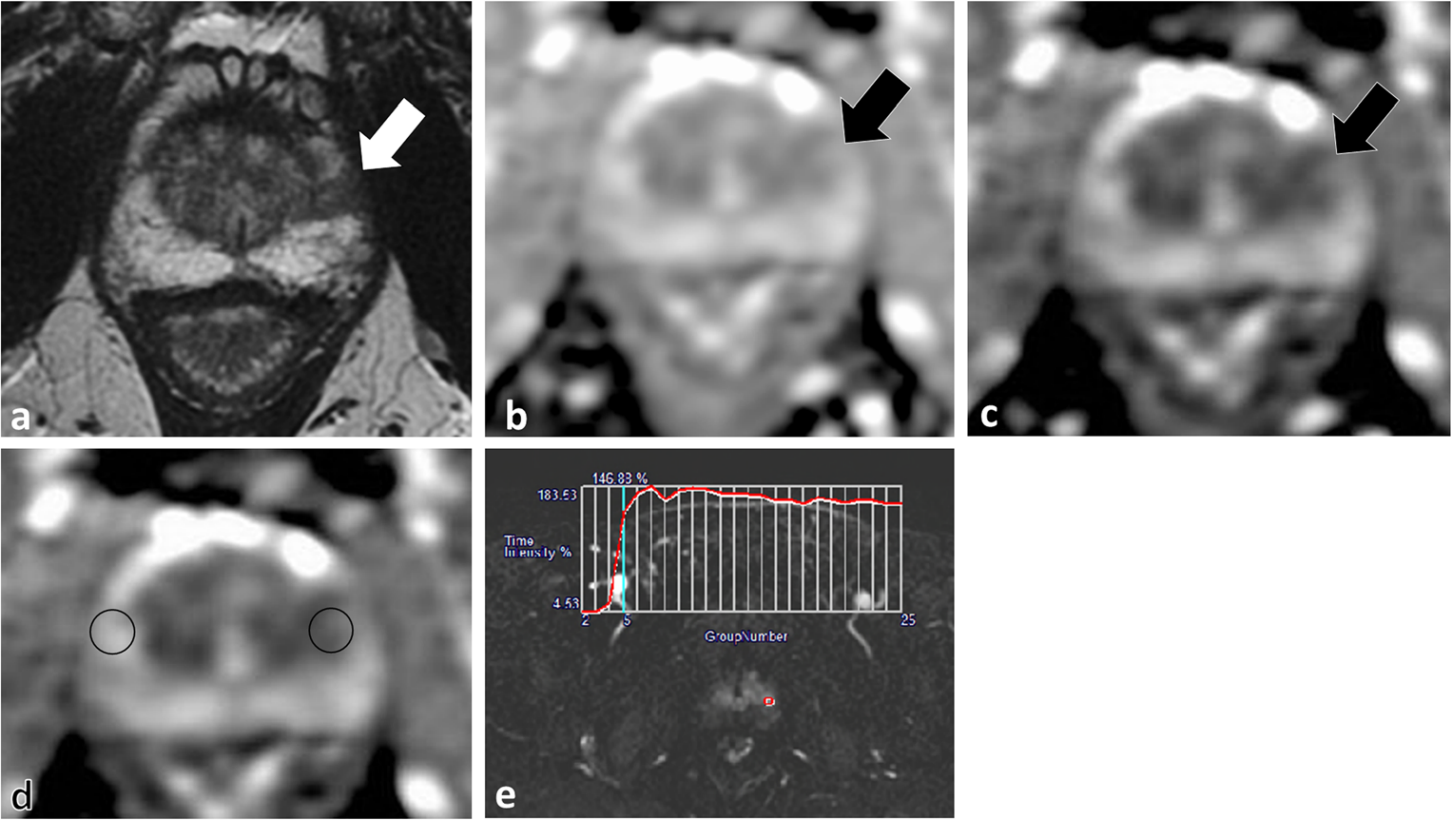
A 55-year-old patient with low-volume Gleason score 3 + 3 = 6 tumour at TRUS biopsy in the left apical PZ underwent MRI prior to consideration for potential AS. Axial T2W TSE image (a) demonstrates a low T2 SI focus in the left apical PZ (*white arrow*). Axial ADC map displayed with automatic windowing/levelling shows minimal decreased SI (*black arrow* in b). These findings could be considered typical for a 3 + 3 = 6 cancer. With modified display of the ADC map using previously validated settings (width = 1.650 and level = 1.675 × 10–6 mm²/s) the nodule (*black arrow* in c) is noted to be of lower SI than initially displayed in (b). Using quantitative data, the ADC value obtained within the tumour (d) was 1.462 × 10^−3^ mm²/s, which would also be considered to be of low (Gleason score 6) grade using previously reported thresholds. Comparing ADC values across systems is challenging due to a lack of standardization, and an ADC ratio has been previously proposed as a better metric to compare ADC. An ADC value from the contralateral normal PZ obtained at the same level was 2.000 × 10^−3^ mm²/s, which yields an ADC ratio of 0.73, which would be compatible with a Gleason score ≥7 tumour based on previously published thresholds. Corresponding DCE image (e) from the same level demonstrated a focal enhancing nodule with a type III contrast curve. Based on the imaging findings, a repeat TRUS-guided biopsy was performed, which demonstrated Gleason pattern 4 in the left apical PZ
